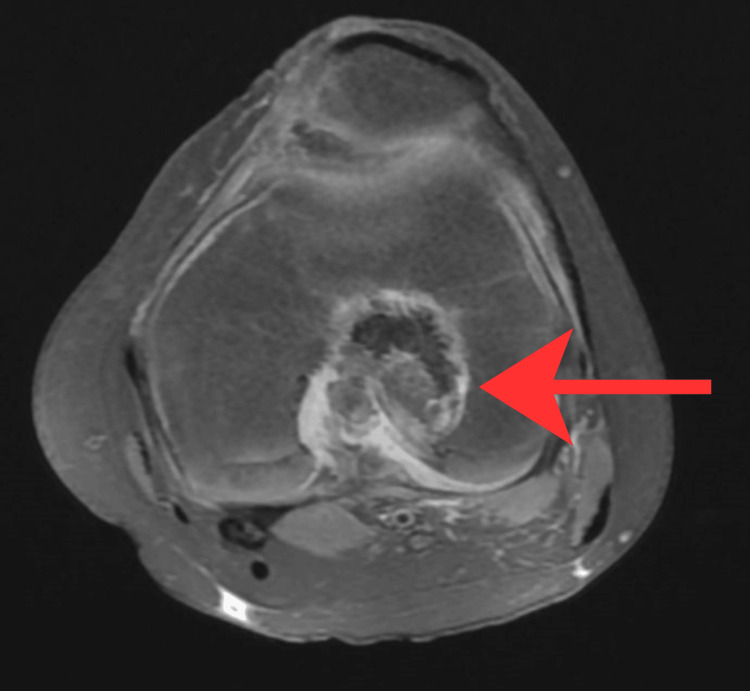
Immediate postoperative MRI of the left knee. Mucoid degeneration of the anterior cruciate ligament and significant dystrophic calcification within the posterior femoral intercondylar notch (red arrow). MRI, magnetic resonance imaging
